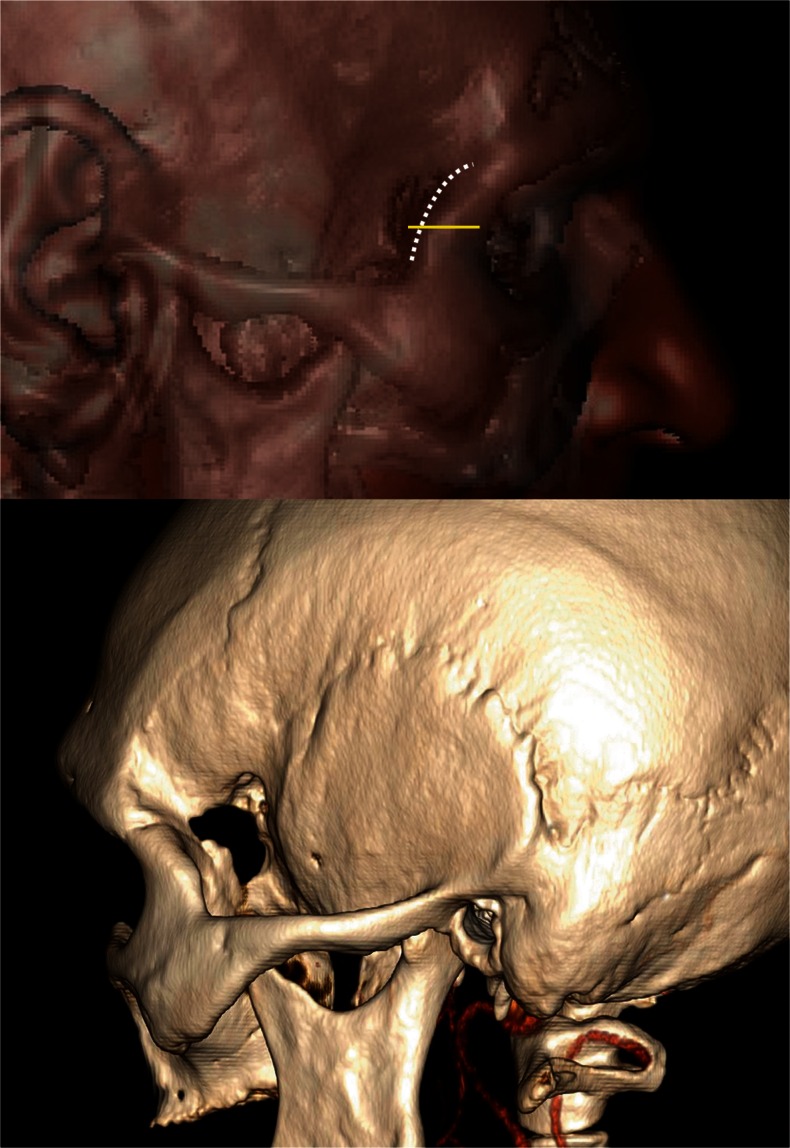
Postoperative 3D computed tomography (CT) scan showing the localization and extent of lateral orbitotomy with marked incisions: skin, horizontal line and temporal fascia, dotted line
